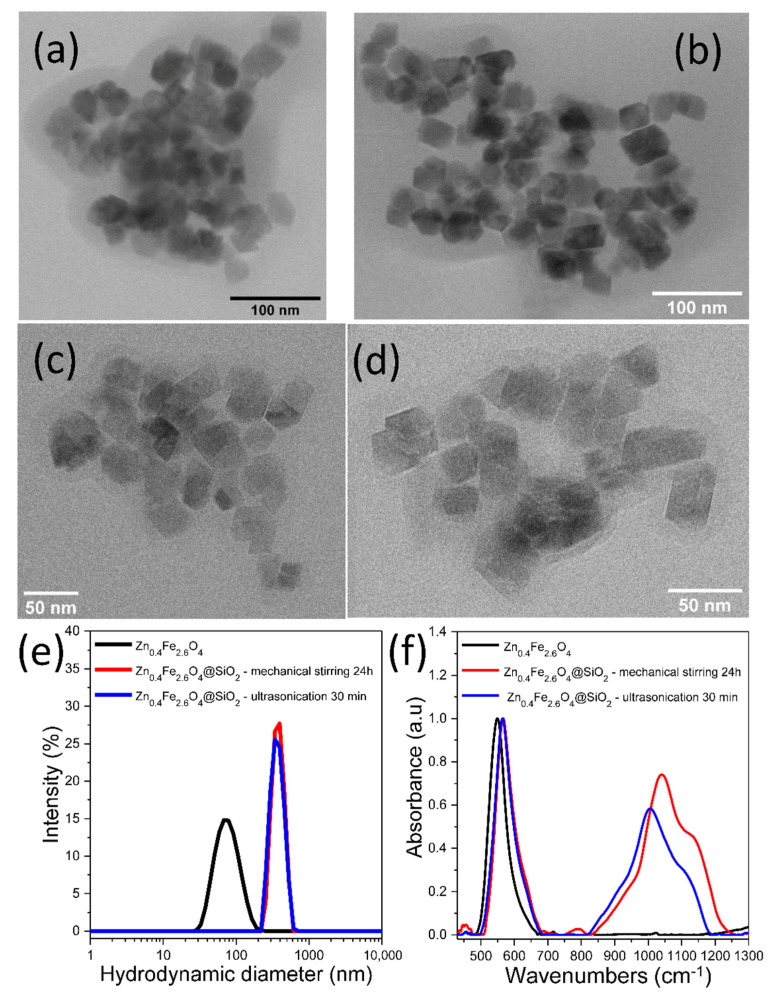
TEM images of Zn_0.4_Fe_2.6_O_4_@SiO_2_-W clusters made via *w*/*o* microemulsion using (a,b) mechanical stirring for 24 h and (c,d) ultrasonication for 30 min. (e) Hydrodynamic diameter resulted from DLS measurements of uncoated Zn_0.4_Fe_2.6_O_4_ and Zn_0.4_Fe_2.6_O_4_@SiO_2_-W clusters dispersed in water at a concentration of 10 μg_MNPs_/mL. (f) FT-IR spectra of uncoated Zn_0.4_Fe_2.6_O_4_ and Zn_0.4_Fe_2.6_O_4_@SiO_2_-W clusters. The spectra are normalized to the highest absorption band.
